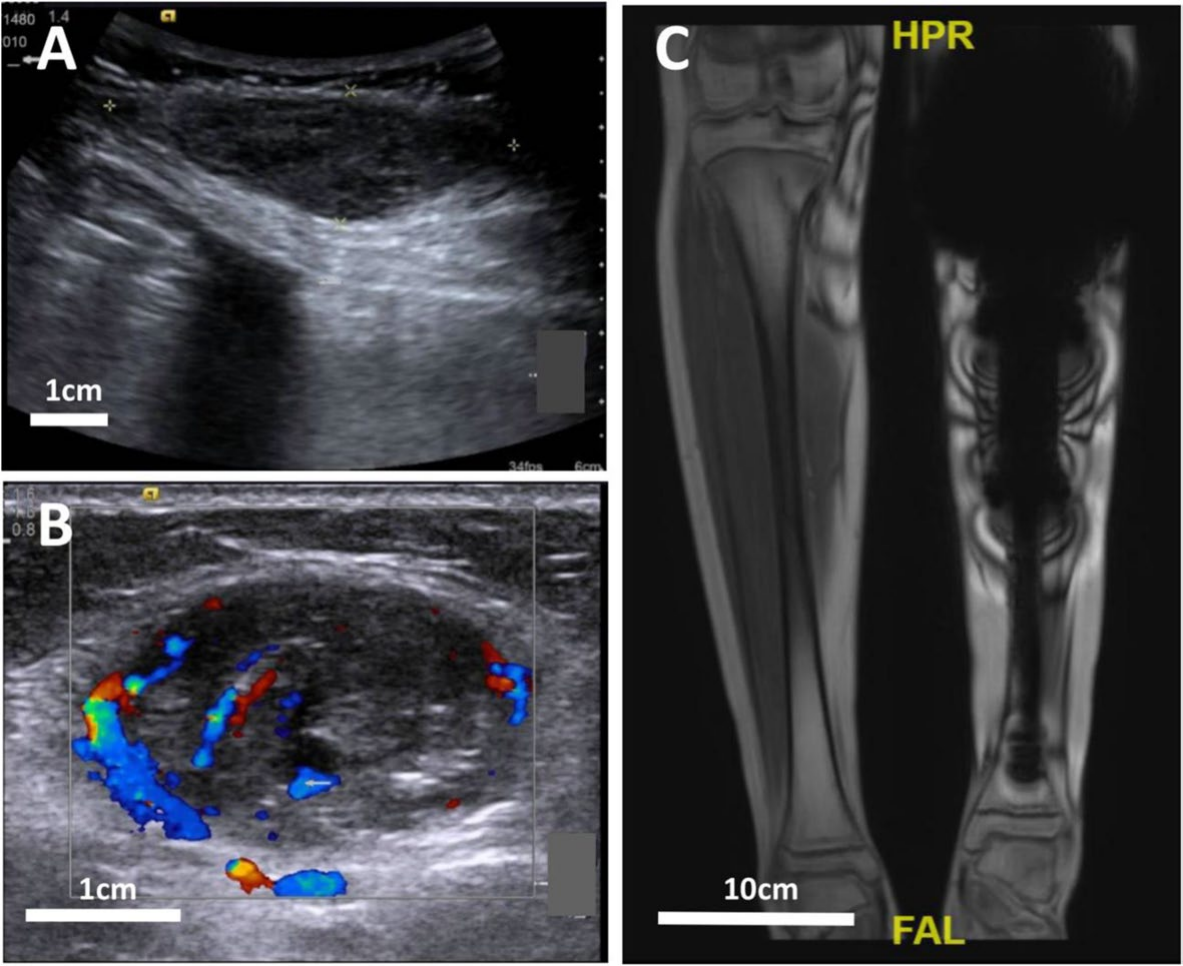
Comparative imaging findings demonstrating lesion visibility on US but not MRI. A US image revealing a hypoechoic mass in the left leg's postoperative soft tissue. B Additional US image showing a vascularized mass within the lateral gastrocnemius. C MRI T1-weighted image obscured by metal prosthesis artifacts, preventing lesion visualization
